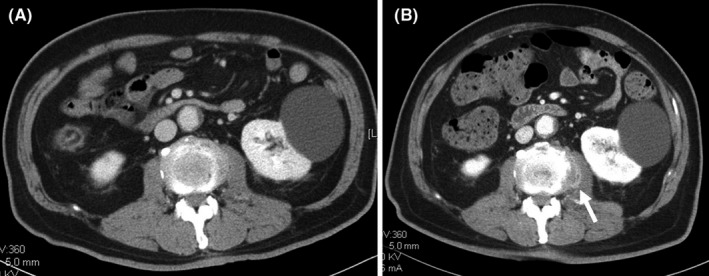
A, A contrast CT scan on admission. Abscess was not detected. B, A contrast CT scan on day 15. Psoas abscess was seen (white arrow)
